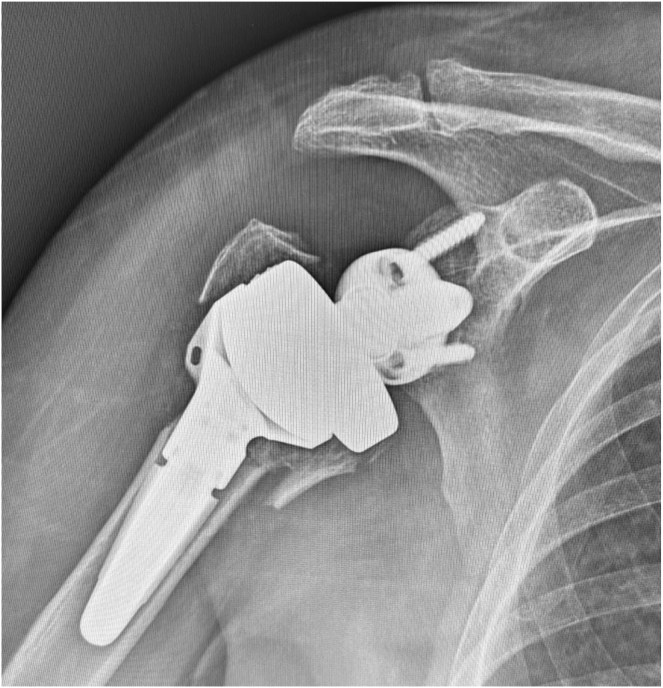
Follow-up radiograph of a *Right* shoulder (86 year old) at 12 months postoperatively, showing nonunion of the tuberosities with migration.
